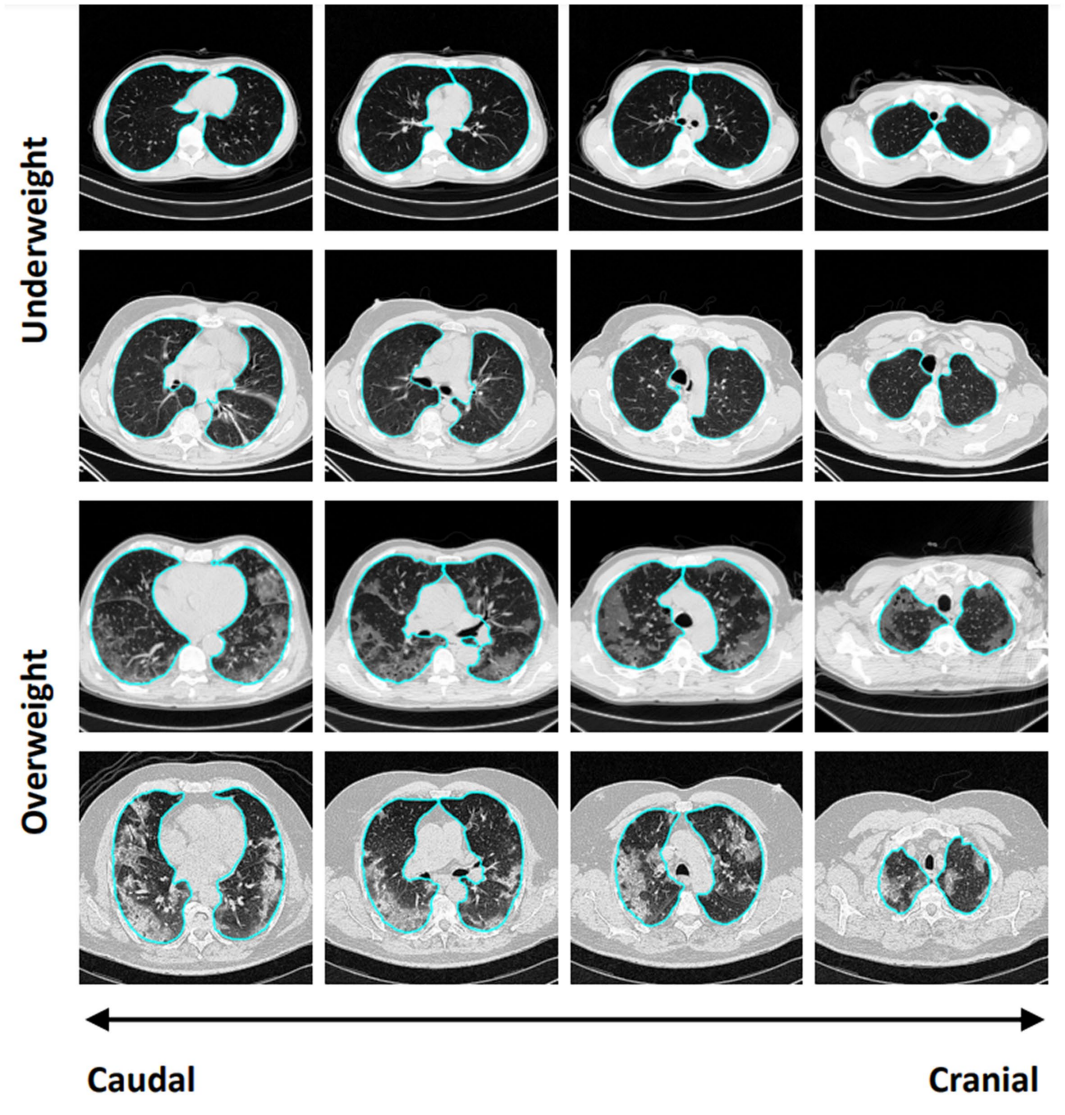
Representative CT images, from cranial to caudal, from two patients with underweight, defined by ≤0% of excess lung weight, and two patients with overweight lungs, defined by >0% of excess lung weight.
